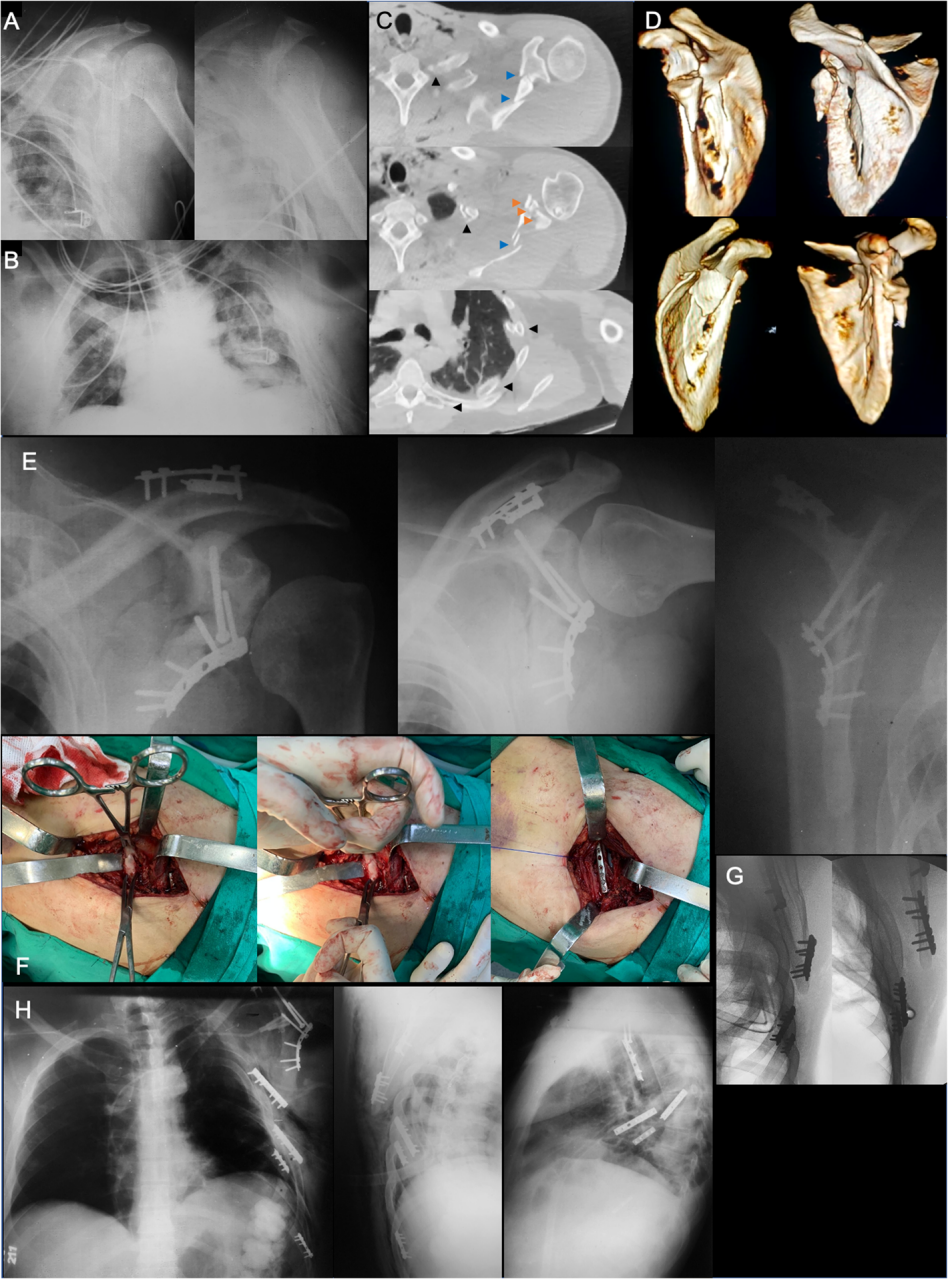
a: Preoperative AP and lateral scapular radiographic views of the left shoulder of a 42-years-old male polytraumatized patient done in the Intensive Care Unit (ICU), showing a comminuted displaced complex scapula fracture; b: Anteroposterior (AP) radiograph of the thorax done in the ICU, demonstrating a drain tube in the left hemithorax due to a traumatic haemopneumothorax; c: Preoperative CT axial cuts of the left shoulder and hemithorax, demonstrating the comminuted displaced complex scapula fracture, involving fragmentation of the glenoid fossa (orange arrowheads) and the scapular body (blue arrowheads). Note the multiple contiguous displaced rib fractures (black arrowheads), extending from the 3rd to the 9th left rib; d: Preoperative 3-D CT reconstructions showing comminuted displaced complex scapula fracture, involving fragmentation of the glenoid fossa and the scapular body. Observe the angled fracture of the spine of the scapular; e: Immediate postoperative true AP, AP, and lateral scapular views of the left shoulder demonstrating the fixation of the most proximal fractures of the scapula. Note the anatomic reduction of the glenoid fossa fracture. Patient was operated on in two steps, apart 5 days from each other; f: Intraoperative images of the 2nd operative procedure performed for the management of some rib fractures and the inferior angle of the scapular body. Observe the sequential reduction and fixation of the 6th left rib with a 2.0-mm straight non-locked plate; g: Intraoperative fluoroscopic images demonstrating the final fixation of the 6th, 7th, and 9th rib fractures, and the inferior angle of the scapular body; h, Postoperative AP, oblique, and lateral radiographs of the thorax, demonstrating the adequate reduction of both the complex left scapular and the multiple left rib fractures. Postoperative in-hospital and after discharge management protocols are the same as previously described for glenoid cavity fractures
